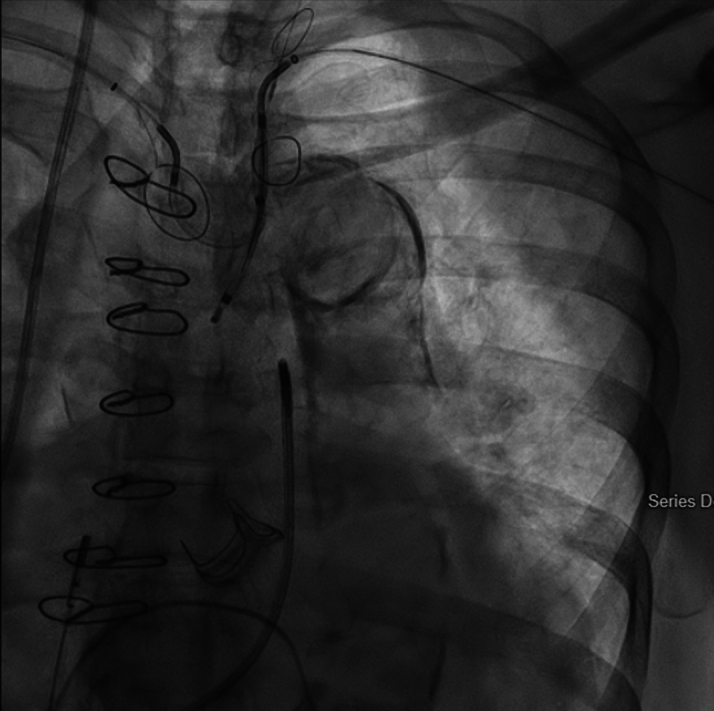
Radiographic Projection Demonstrating the Position of 2 SENTINEL Cerebroembolic Protection Devices With Baskets Deployed in the Brachiocephalic Trunk, Left Common Carotid Artery, and Left Subclavian Artery The right-sided device was introduced via the right ulnar artery and deployed in the usual fashion. The left-sided device was introduced via the left ulnar artery. The proximal basket was then deployed within the left subclavian artery, and the distal basket was left undeployed.
